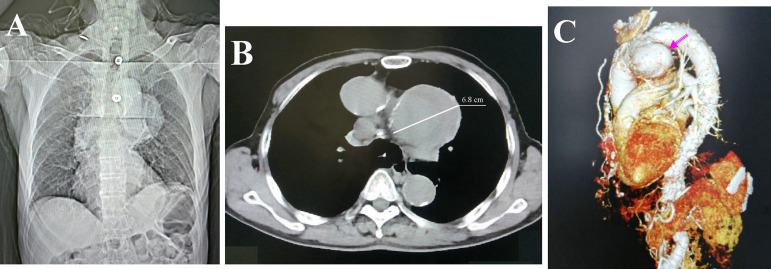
Computed tomography (CT) of an aortic arch pseudoaneurysm in a 73-year-old male patient presenting with a two-week hoarseness. (A) The first frame imaging of CT scan showed a protruding aortic arch into the left upper thoracic cavity; (B) an axial view of the aortic arch pseudoaneurysm measuring 6.8 cm in diameter; and (C) the aortic arch pseudoaneurysm arising from the inferior aspect of the arch on three-dimensional CT.
